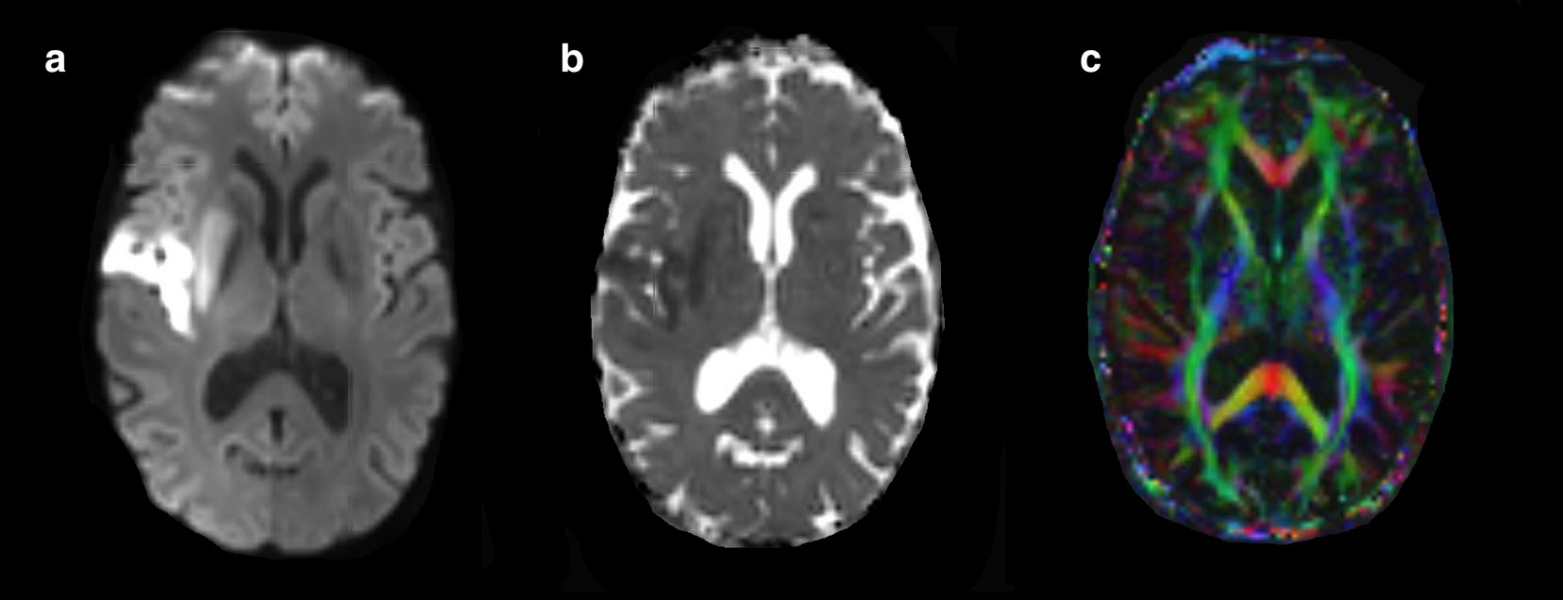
Diffusion‐weighted imaging (DWI) of the brain (a) with the corresponding apparent diffusion coefficient (ADC) map (b) and diffusion tensor imaging (DTI) map (c). Restricted diffusion due to right‐hemispheric ischemic stroke (affecting the insular region as well as putamen) in (a) is visualized as hyperintense signal. Corresponding ADC signal decreases in (b) are shown in grayscale. In (c), the orientation of white matter fiber bundles is shown within the brain by assigning a principal color component (red, green, and blue) to a principal direction (left–right, anterior–posterior, and superior–inferior), referred to as directionally encoded color (DEC). Although widely used, good visibility for people with impaired color vision is difficult for DTI data with DEC, given that it needs three color coordinates to convey information on a three‐dimensional vector.
